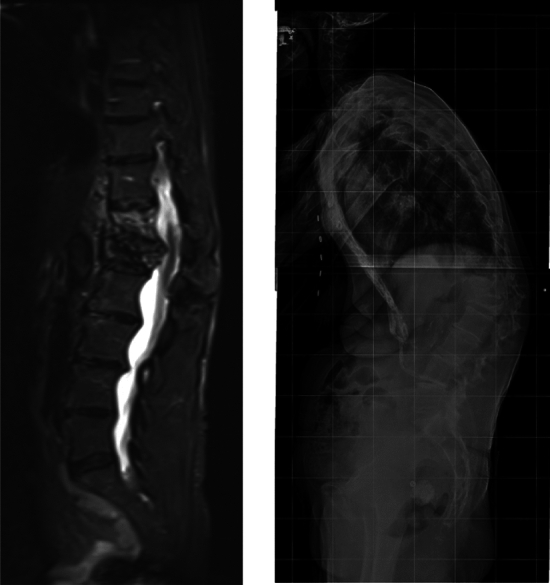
A MRI showing T12 and L1 burst fractures in a recumbent position. B standing full-spine radiograph showing massive kyphotic collapse of the fracture despite brace therapy
